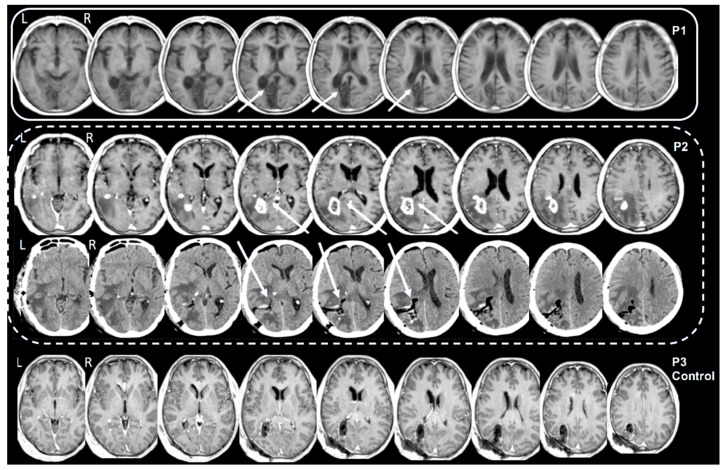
Patient 1 (P1) had a lesion that included the visual cortex and the forceps major continuing in the splenium of the corpus callosum. There was also a lesion in the left superior colliculus and part of the posterior left thalamus. In Patient 2 (P2, upper line), the MRI brain images pre-surgery, showed a large left posterior temporal and parieto-occipital neoplasm including the optic radiation, but the forceps major fibers were intact. Patient 2 (P2 lower line), the CT brain scan post-surgery showed the resection of the neoplasm including the optic radiation and the forceps major. The arrows indicate the brain region of the forceps major. For Patient 3 (P3 Control), the MRI brain images present a left posterior temporal and parieto-occipital resection of the neoplasm including the optic radiation, sparing the forceps major fibers. Note that none of the patients had lesions in the right hemisphere. L: left hemisphere; R: right hemisphere.
